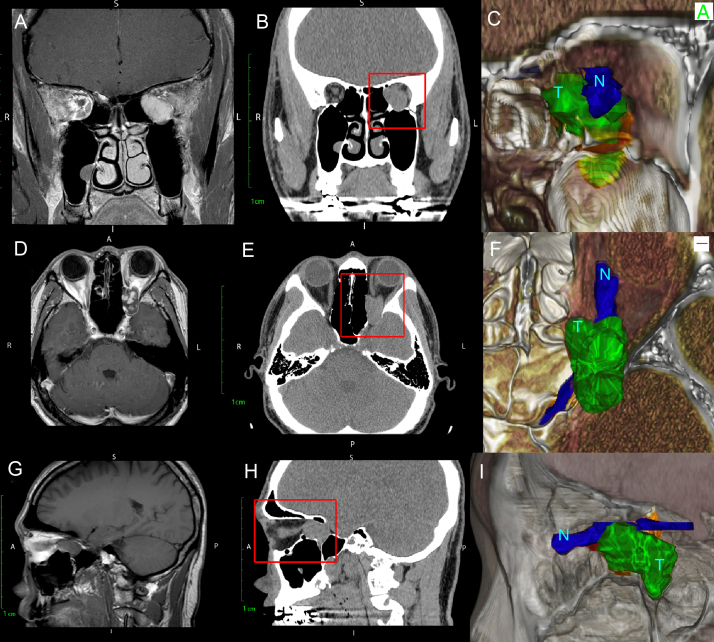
Comparison of T1-weighted MRI (A, D, G) with CT scan (B, E, H) and with 3D rendered (C, F, I) of Patient 1 with a massive orbital tumor. Note how the three-dimensional rendering gives depth to the image and improves the distinction between the ON (N) and orbital Tumor (T) which cannot be fully distinguished on either CT or MRI.
